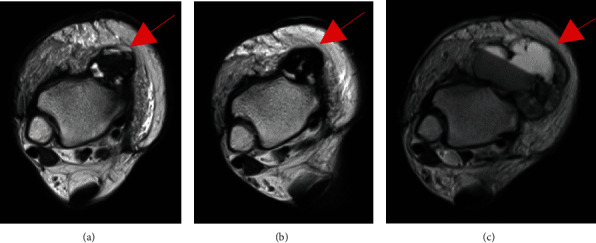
Tumor progression of the local recurrent tumor during tocilizumab treatment. (a) Axial image of the recurrence tumor in the ankle region acquired using T2-weighted MRI at the time of recurrence. (b) Axial image of the recurrence tumor in the ankle region acquired using T2-weighted MRI 2 months after starting on tocilizumab treatment. (c) Axial image of the recurrence tumor in the ankle region acquired using T2-weighted MRI 4 months after the first evaluation. The tumor is indicated by the arrows in red.
